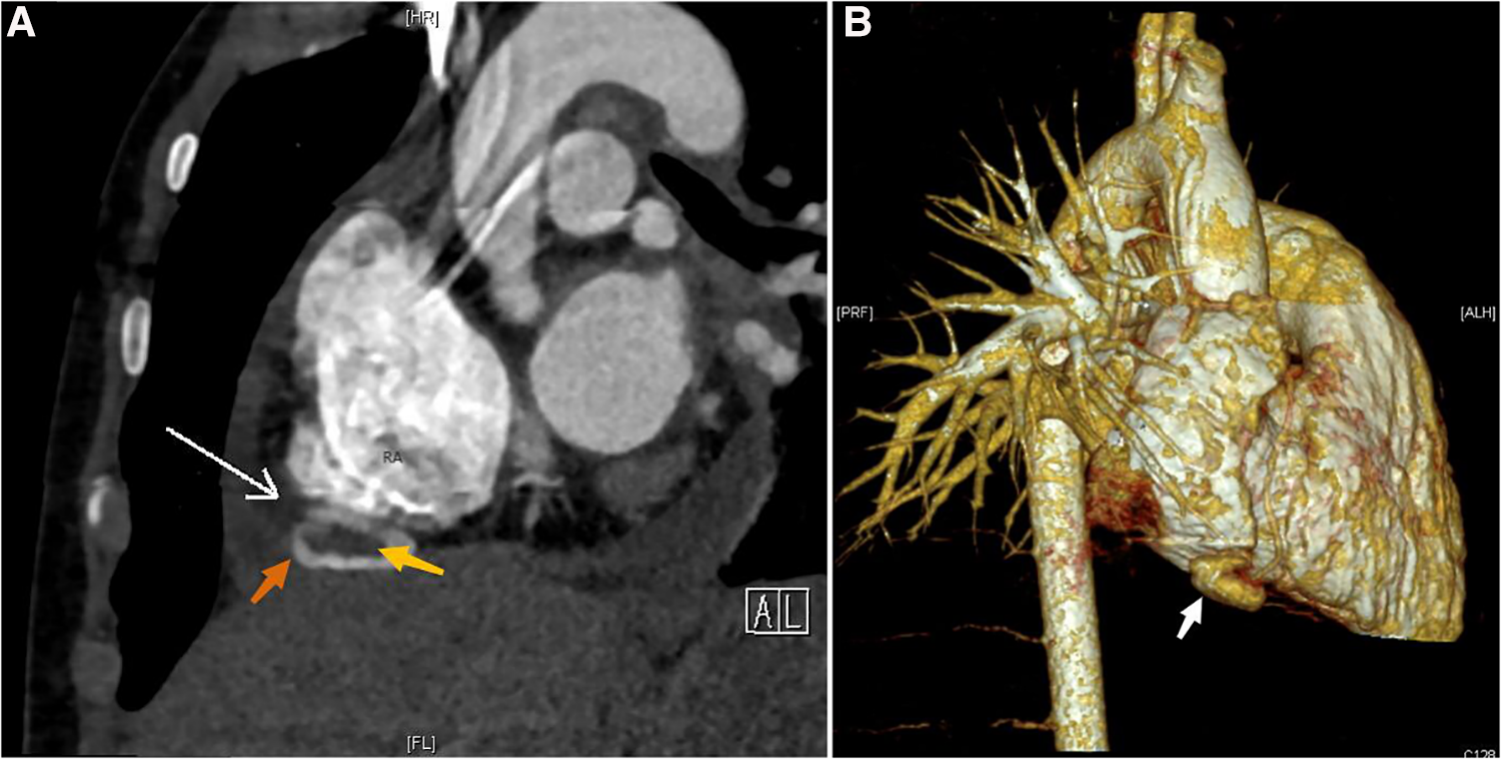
CTA and CT 3D reconstruction: (A) RAA (indicated by the orange arrow), RAA tumor neck (indicated by the white arrow), thrombus (indicated by the yellow arrow); (B) RAA (indicated by the white arrow).
